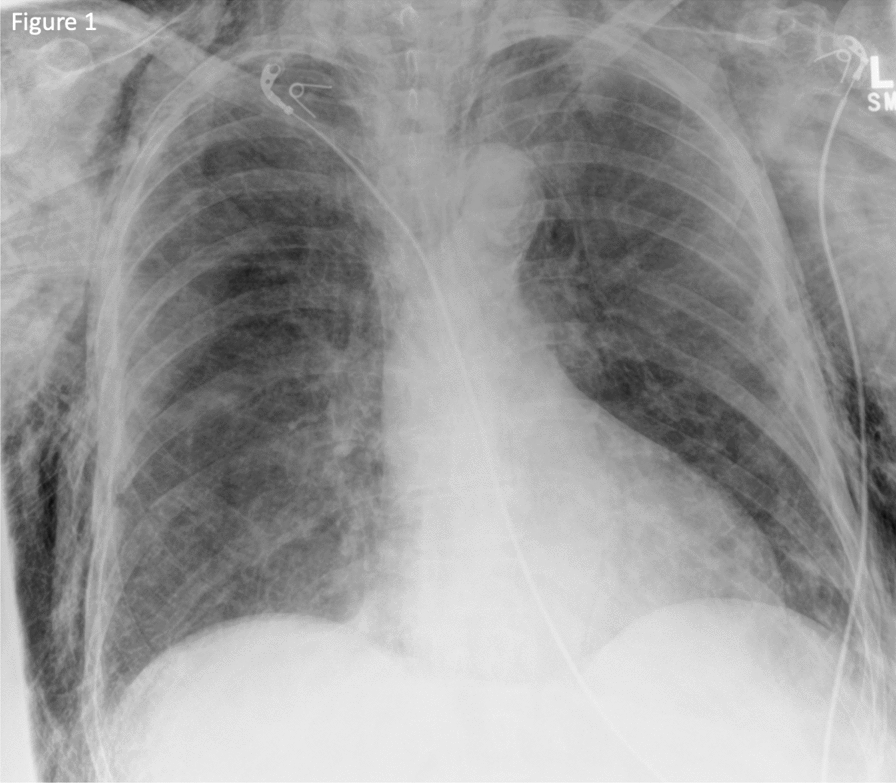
An anteroposterior portable chest X-ray taken in the resuscitation room post-intubation, demonstrating severe subcutaneous emphysema. Pneumothoraces and are not appreciable in this image, due to overlying emphysema
